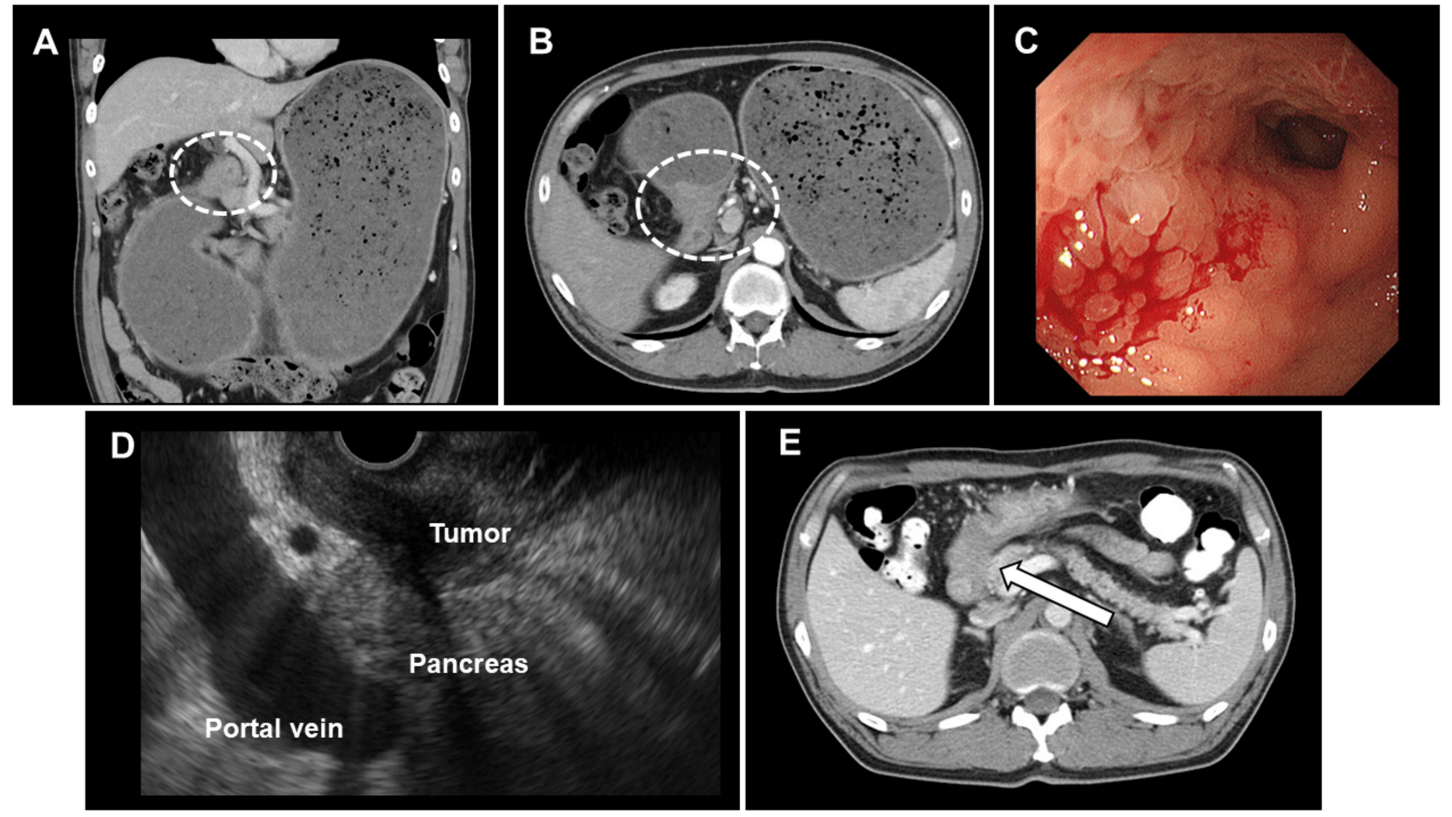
Pre-treatment imaging and endoscopic findings Initial contrast-enhanced abdominal computed tomography (CT) in the coronal (A) and axial (B) planes demonstrates significant gastric residue and pyloric wall thickening (dashed lines). Initial endoscopy (C) reveals a circumferential type 3 tumor near the pylorus, causing pyloric stenosis, marked gastric retention, and duodenal extension. Endoscopic ultrasound (D) shows a large, hypoechoic area in the stomach and duodenum with indistinct pancreatic head borders. Post-decompression CT (E) depicts extensive tumor involvement from the pylorus to the duodenal bulb, with suspected pancreatic head invasion (white arrow).
